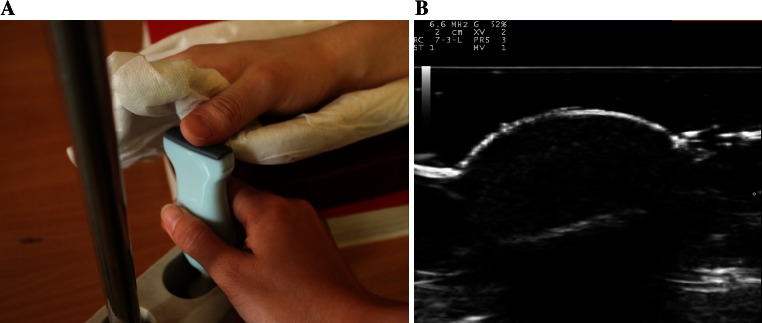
a A finger plaster cast made with gypsum for fixing thumb of the right hand of examinee and the transducer was put in the transducer holder with surface of transducer facing up to thumb of the right hand of examinee. The thumb of the right hand of examinee was gently facing down and putting on the surface of the transducer which was perpendicular to the axis of the thumb. The transducer inside the transducer holder was shifting by operator. b The transducer should touch the surface of the thumb softly to keep the arch of the surface of the thumb on two-dimensional image of ultrasound. The long-axis of the acral arterioles was applied to keep the angulation between sound beam and blood flow ≤60°
